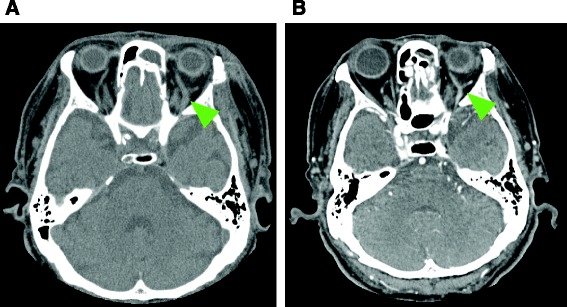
Follow-up computed tomography scan of the superior ophthalmic vein thrombosis. A plain scan was conducted on day 17 (a) and a contrast-enhanced scan was performed on day 17 (b). a The *yellow-green arrowhead* shows that the dilatation of the superior ophthalmic vein had improved (to 4.3 mm). b The *yellow-green arrowhead* shows that the dilatation of the superior ophthalmic vein had improved further (to 3.3 mm) and that the thrombosis had almost been resolved
